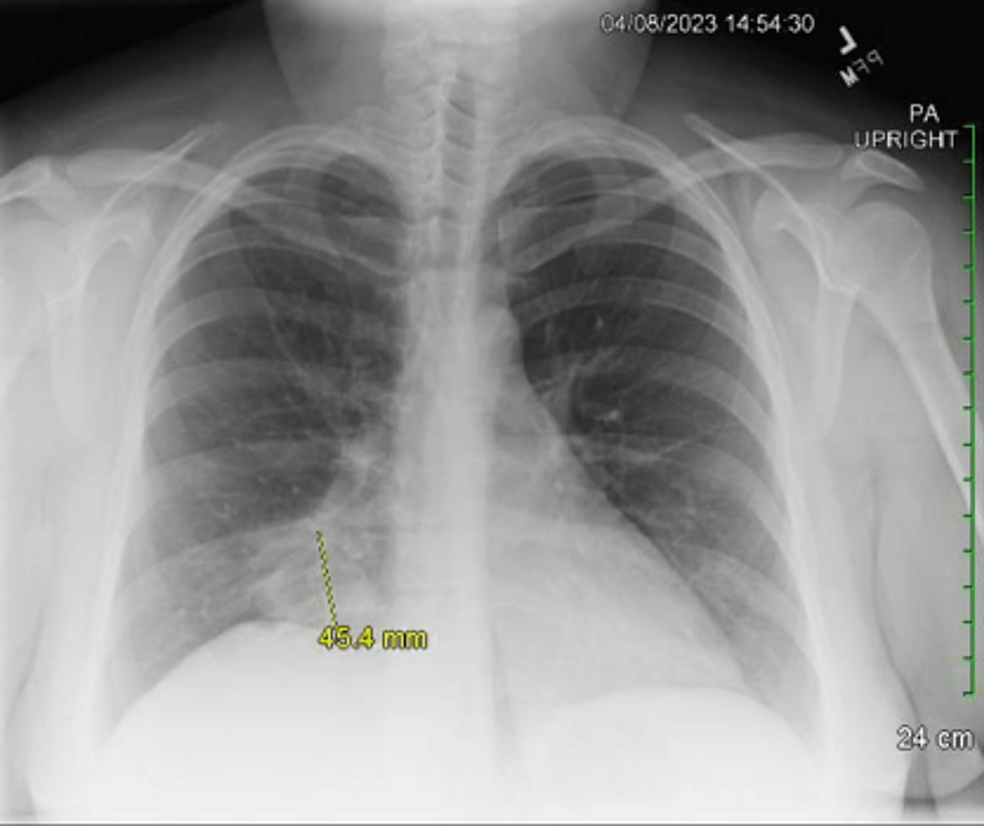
Posterior/anterior chest X-ray from April 2023. The yellow radiology marker depicts the area of interest as well as the marked size.
